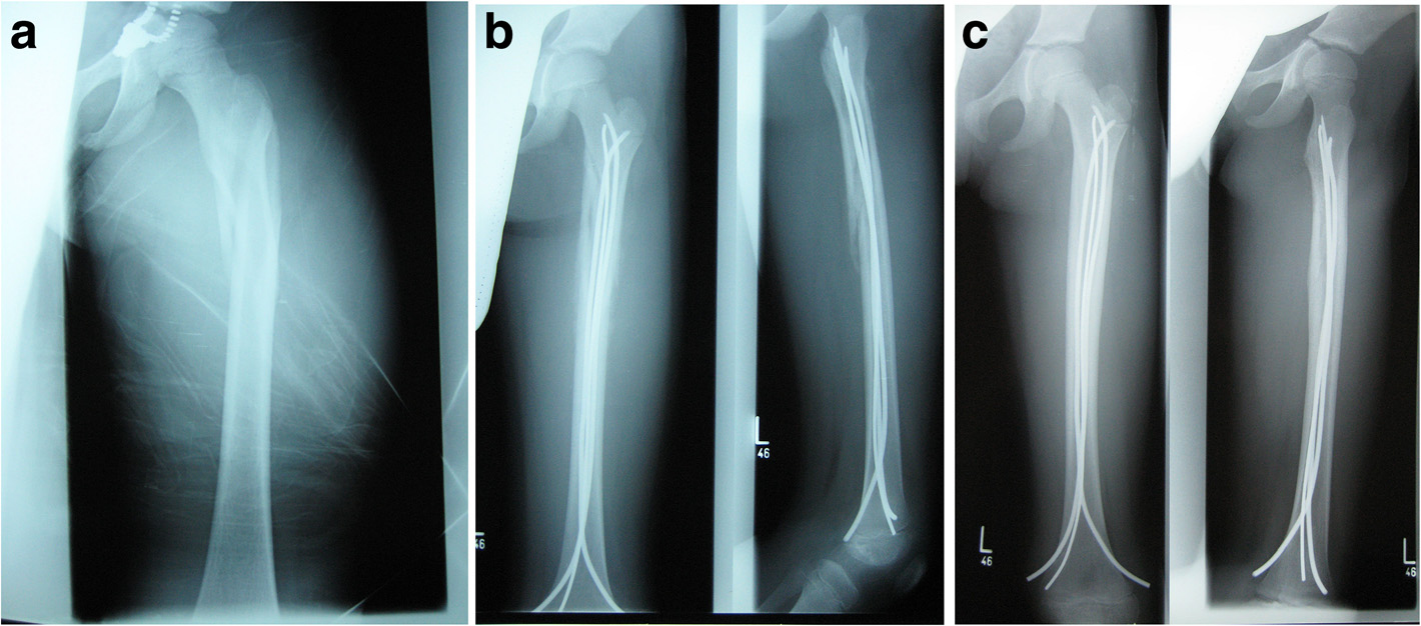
Radiographs of a left femur with a complex proximal spiroid fracture of the shaft. a: Fracture; b: X-Rays a-p and lateral after 1 month, c: X-Rays a-p and lateral after 4 months.
